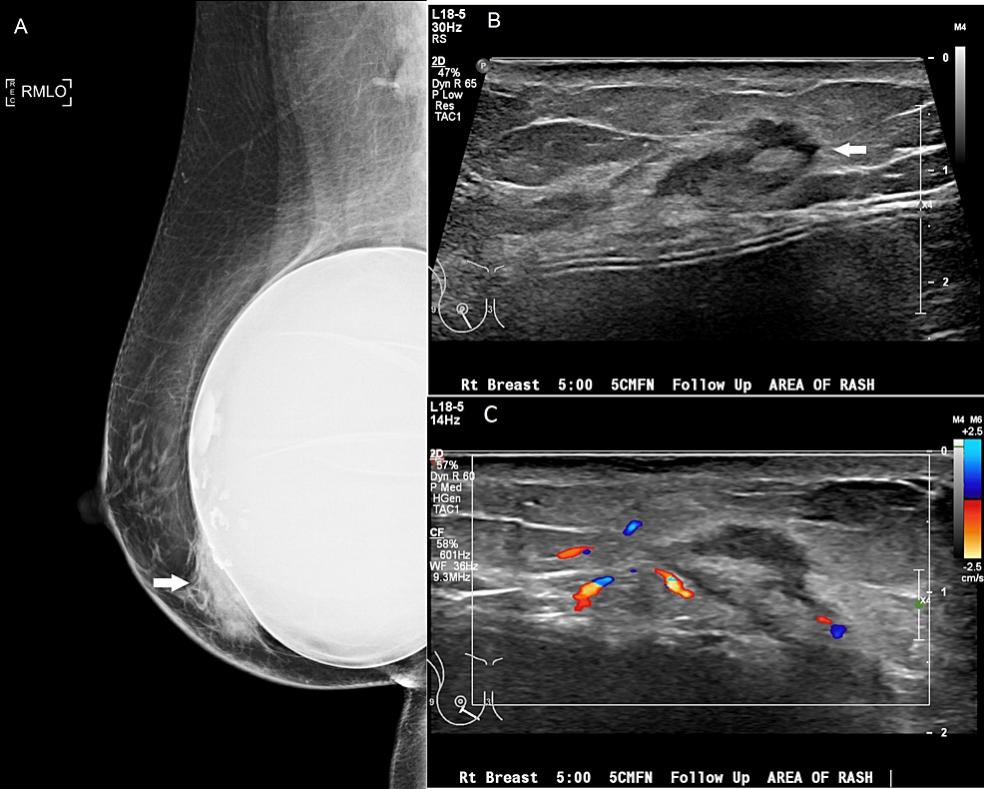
(A) Follow-up mammogram demonstrating an increase in size of the asymmetry (arrow) as well as an increase in skin thickening throughout the inferior breast (compare to Figure 1). (B) Grayscale ultrasound image demonstrating a mixed echogenicity mass (arrow), also increased in size and conspicuity (compare to Figure 1). (C) Color Doppler ultrasound image showing no suspicious vascularity associated with the lesion.
